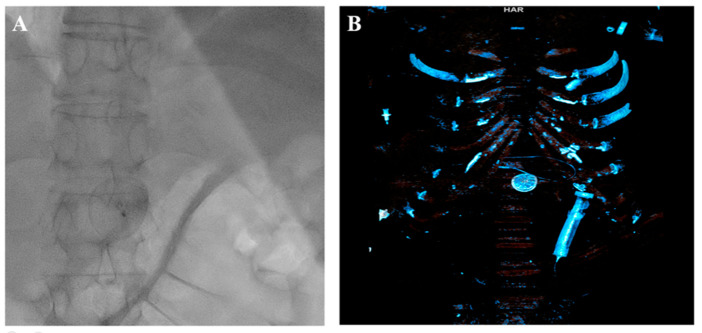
Occluder in the abdominal aorta. (A) Fluoroscopy. (B) Computed tomography.
